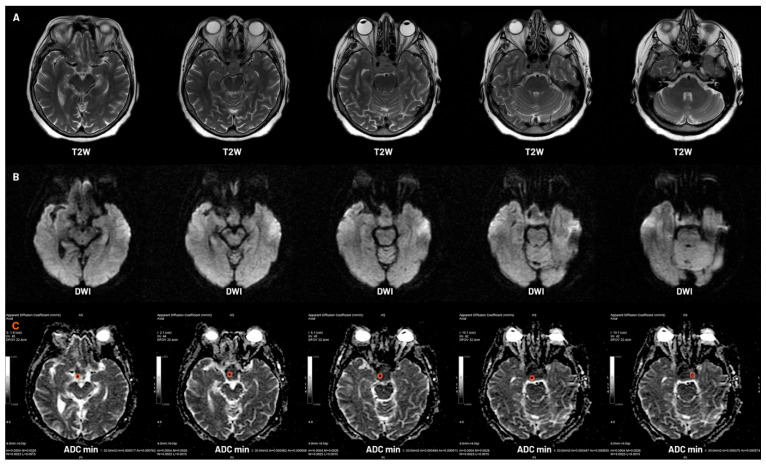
MRI evaluation of a sellar region lesion using conventional and diffusion-weighted imaging. (A) Axial T2-weighted images showing an invasive adenoma. (B) Corresponding diffusion-weighted imaging (DWI) sequences demonstrating restricted diffusion within the lesion. (C) Apparent diffusion coefficient (ADC) maps with ROI-based measurements of ADCmin across consecutive axial slices, indicating areas of lowest diffusivity.
